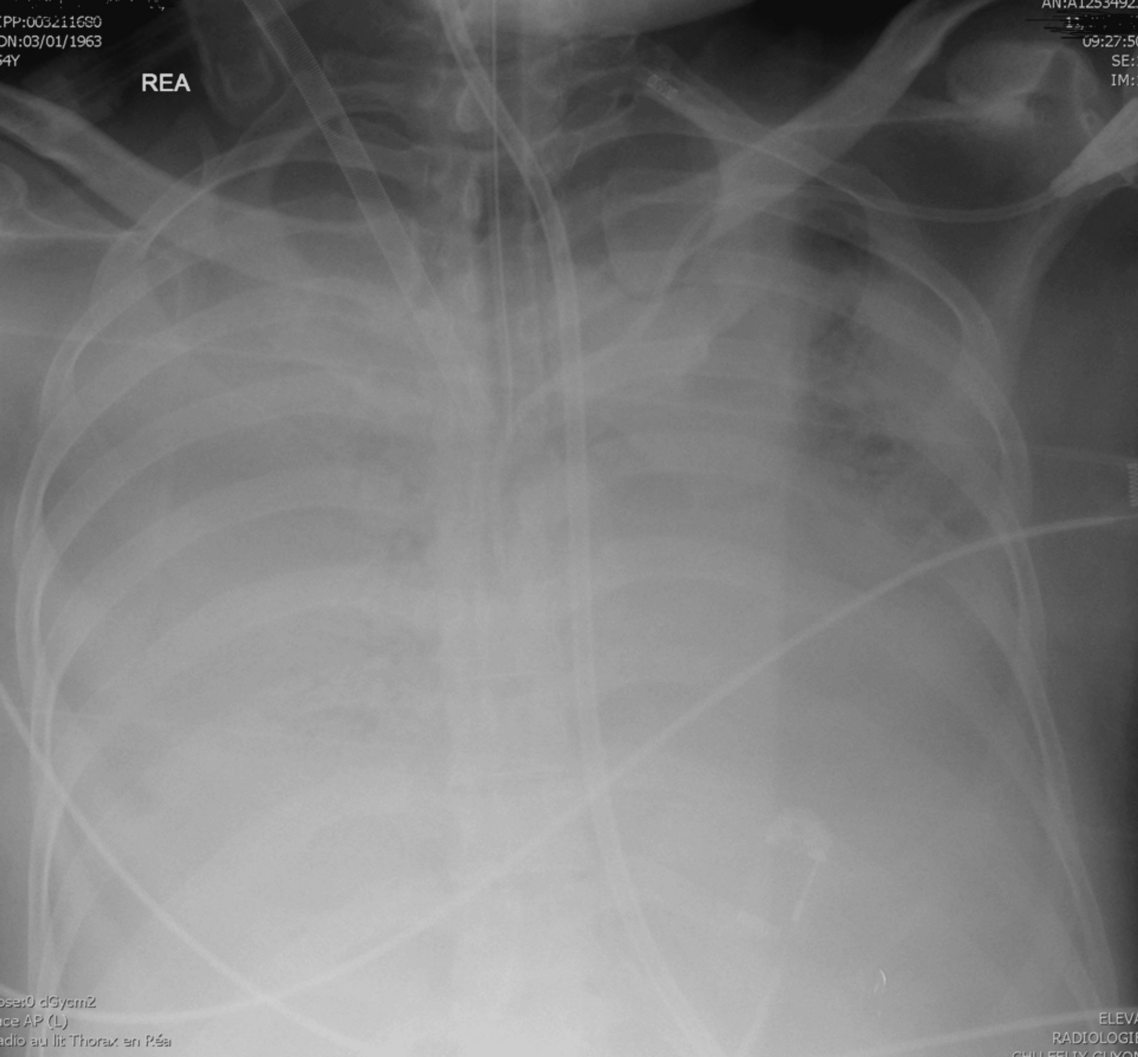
Chest X-ray at the bedside Diffuse alveolar shadows (acute respiratory distress syndrome, ARDS)
